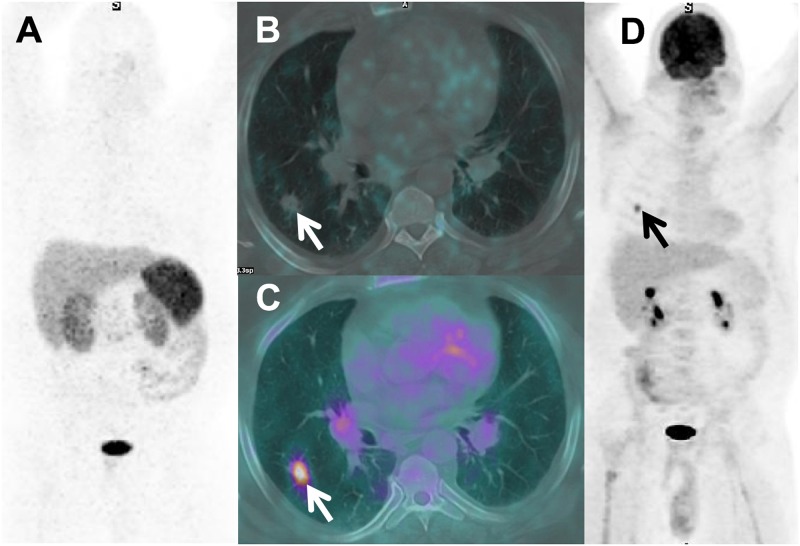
Discordant image, FP ^18^F-FDG, TN ^68^Ga-DOTATATE PET/CT. Axial CT (A) with a spiculated IPN. Fused ^18^F-FDG PET/CT (B) with IPN intense uptake (SUVmax 5.8) vs. ^68^Ga-DOTATATE (C) with no visible uptake (SUVmax 0.90). Comparison ratios of the SUVmax of the nodule to normal lung and aortic blood pool for 18F-FDG were 11.8 and 6.4, respectively, with corresponding values of ^68^Ga-DOTATATE being 2.2 and 1.5. Biopsy revealed inflammatory cells; nodule resolved on CT follow-up.
